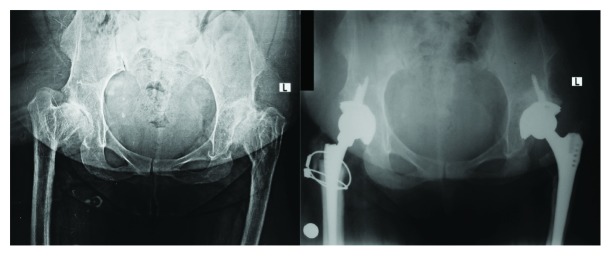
Preoperative and postoperative 2-year pelvis anteroposterior radiographs of the patient.
